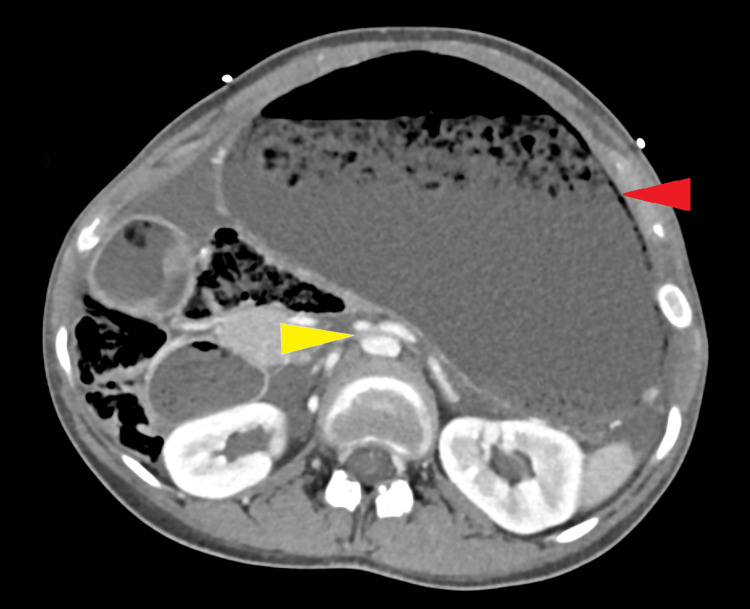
Axial view of the abdominal CT scan showing a reduced AM distance and gastric distention with pneumatosis Yellow arrow: aortomesenteric distance. Red arrow: gastric pneumatosis in the distended stomach. CT: computed tomography; AM: aortomesenteric
